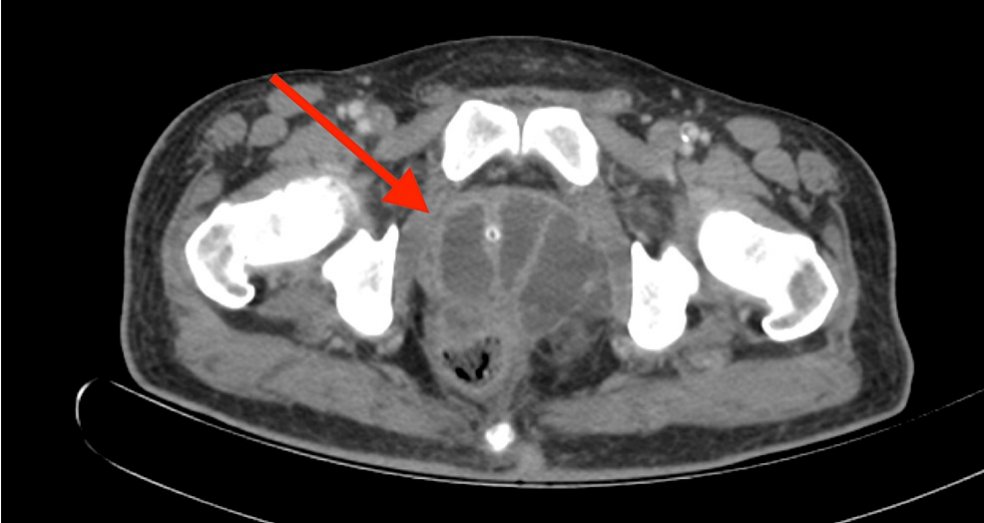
Computed tomography demonstrates multiloculated prostate abscess and transurethral segment of bladder catheter
